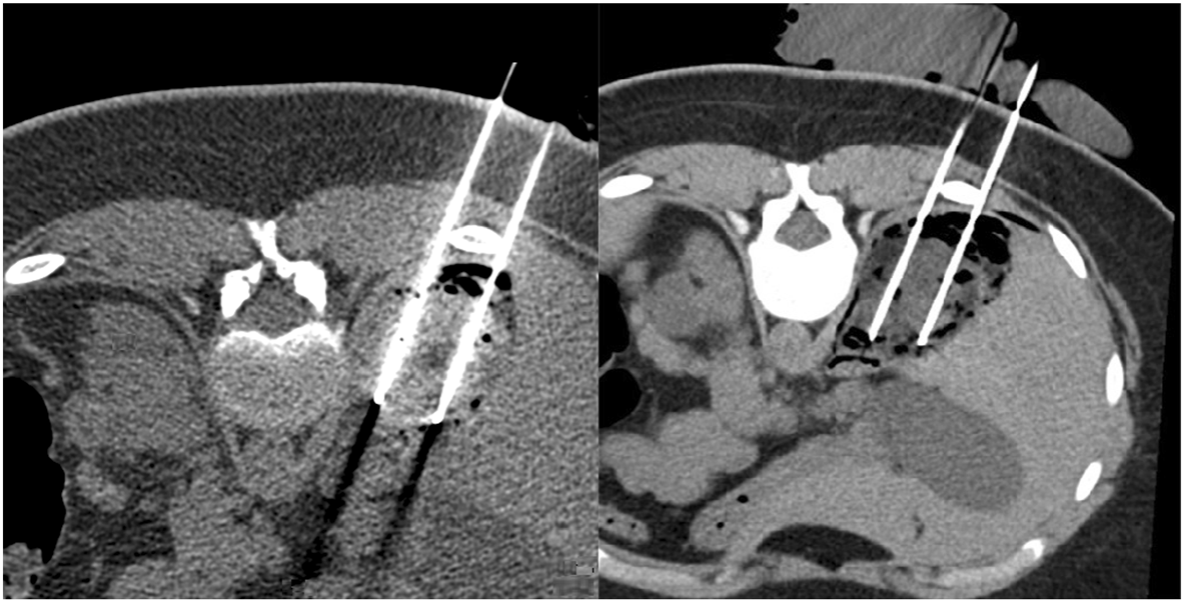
Axial computed tomography (CT) slice showing 2 cryoprobes in the AML. The bowel and the liver has been pneumodissected. Left image is before freezing and right image is after freezing.
